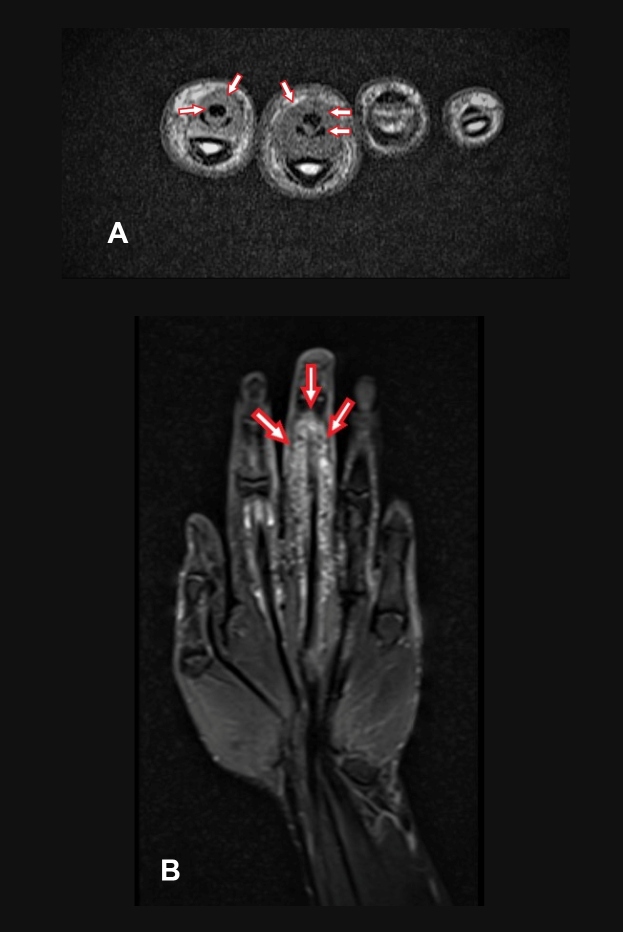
(A) Wrist magnetic resonance imaging. Pre-operation non-enhanced fat suppressed T2-weighted axial and coronal (B) images show multiple hypointense rice bodies in the enlarged synovial fluid around the digital flexor tendon sheaths of the third and index fingers (arrows).
